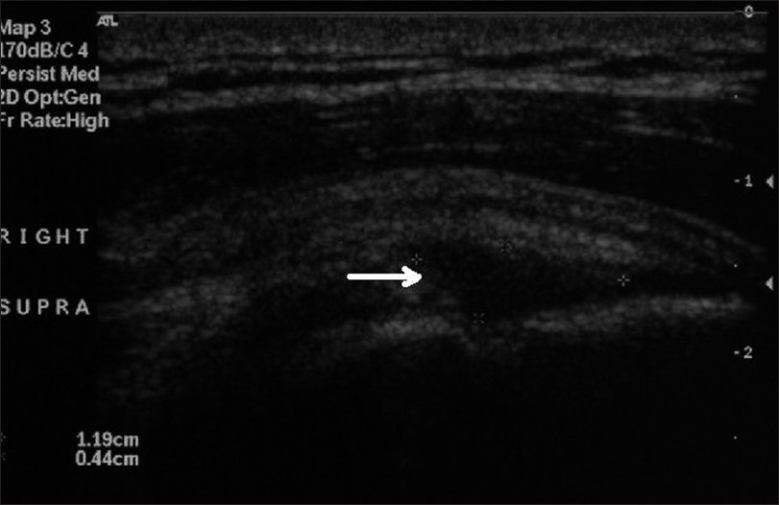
Ultrasound showing partial-thickness rotator cuff tear (arrow), indicated by a distinct hypoechoic defect at the articular surface of the supraspinatus tendon
